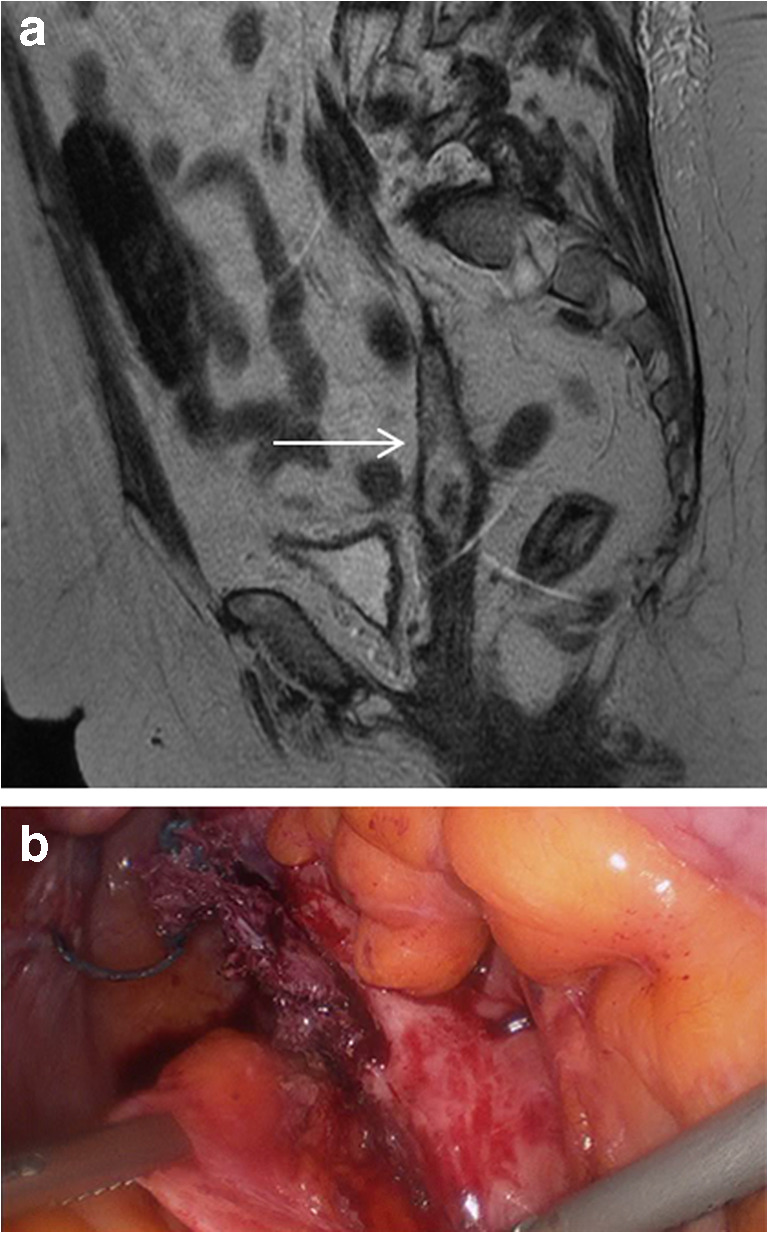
MR scan (A) and corresponding laparoscopic image (B) of an infected mesh tract. A MRI demonstrating an infected mesh tract with vaginal wall erosion (solid arrow) and the corresponding laparoscopic image (B) from the same woman demonstrating the partially excised thickened infected mesh tract
